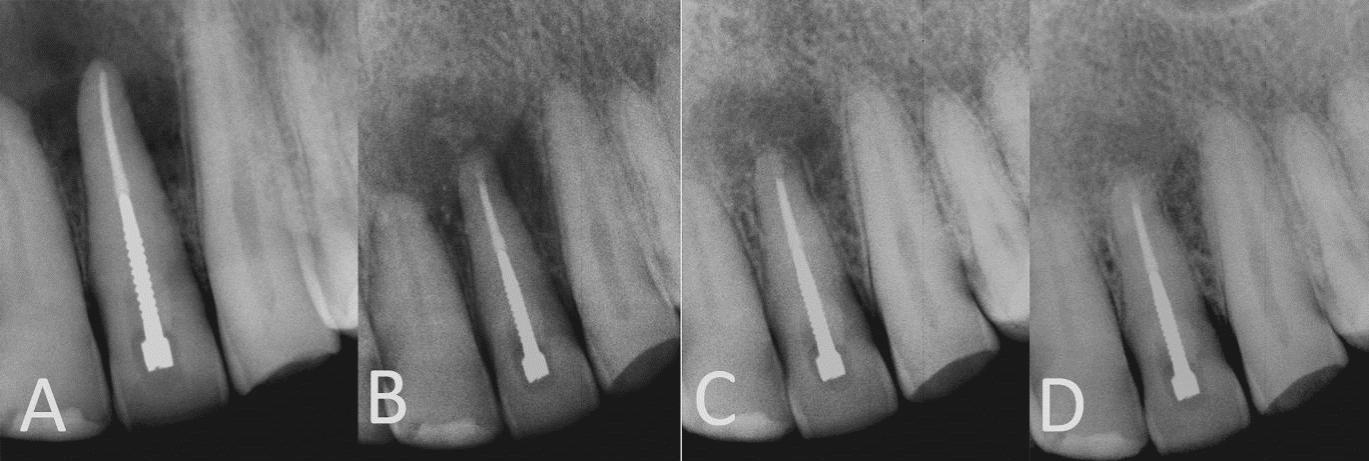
A) A maxillary left lateral incisor with extensive periradicular lesion (case No. 9); B) immediately after periradicular surgery; C) 4 months after surgery; D) one-year follow-up radiograph shows normal PDL
